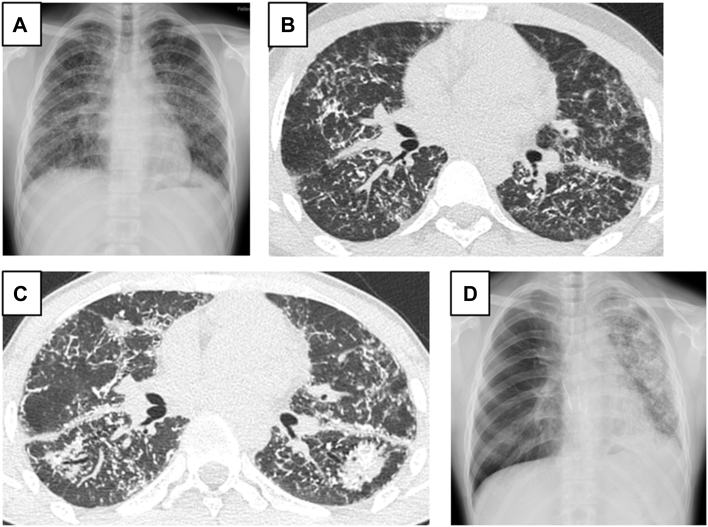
Chest radiography shows bilateral numerous dense nodules (A) and computed tomography shows multiple high-density nodules (B) at evaluation for listing. (C) Chest computed tomography before transplantation shows severe worsening of branching calcifications. (D) Chest radiography performed 35 months after transplantation shows progressive pulmonary ossification of the native lung.
